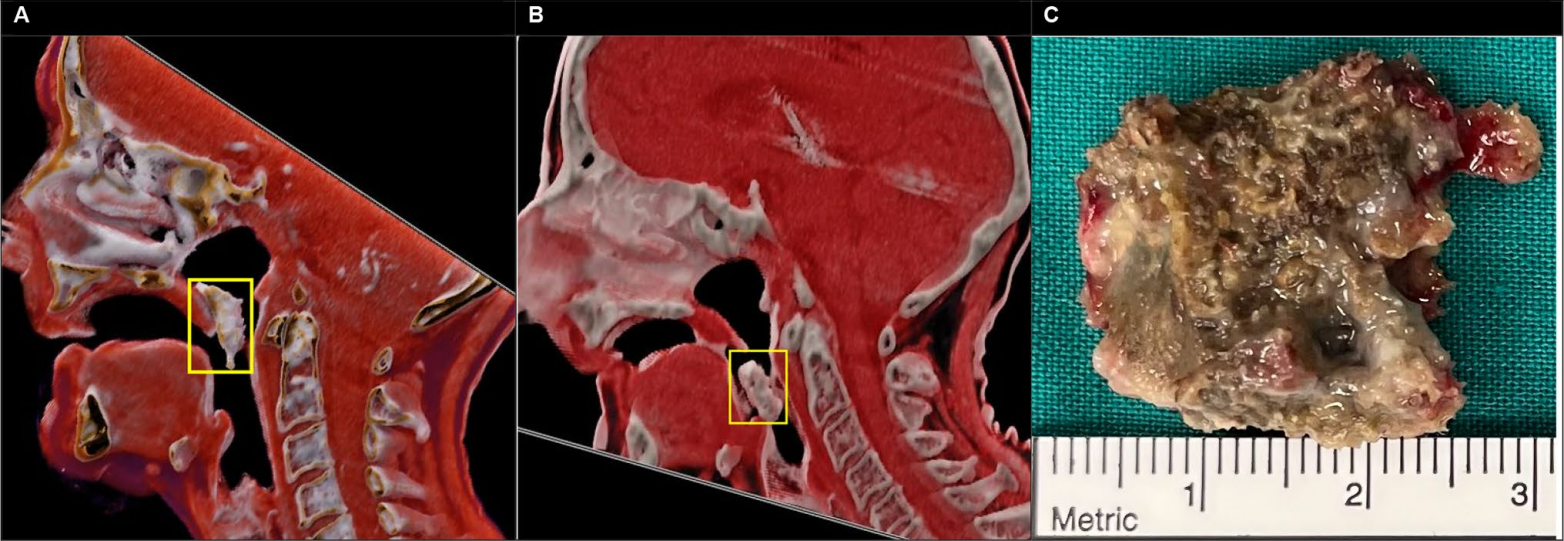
VRT-reconstruction of the CT scans (A on March 10th 2023 and B on March 12th 2023) showing that the dislocation of the fractured clivus caused an almost complete obstruction of the laryngeal inlet within 48 h (A + B). The final picture of the dislocated clival sequestrum after successful transoral removal (C)
